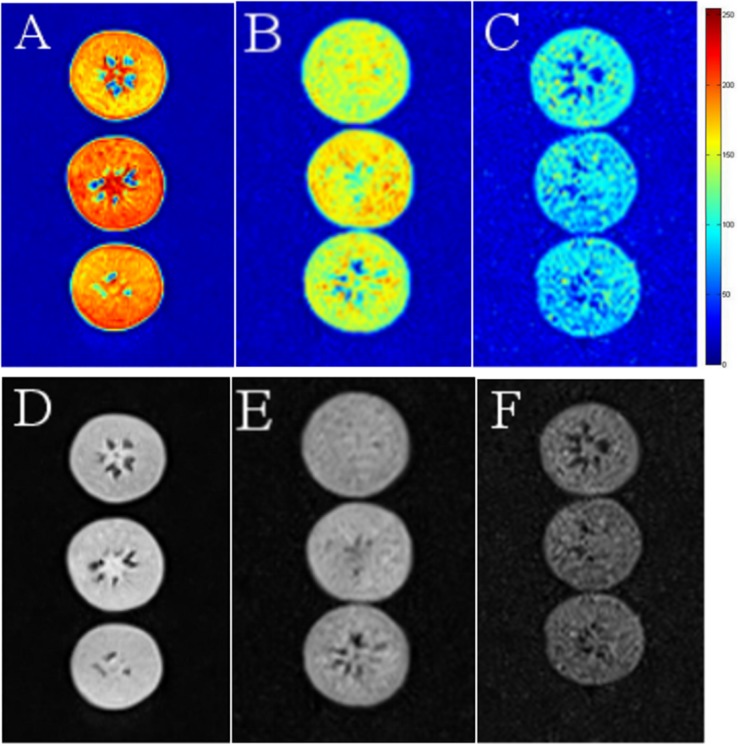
Nuclear magnetic resonance images of fresh (A,D), LT (30 days) (B,E), and LT (30 days) + 4 days (C,F) samples. The images were color coded based on their grayscale value. Red corresponds to the “bright part” and blue to the “dark part.” Fresh is the fruit sample on the day of harvest, LT (30 days) is the fruits cold stored for 30 days, and LT (30 days) + 4 days is the fruits on the fourth day of shelf life after 30 days of cold storage (with pitting). The experiment was repeated three times. To clearly indicate the moisture content in blueberries, a representative set of data was shown in the figure.
